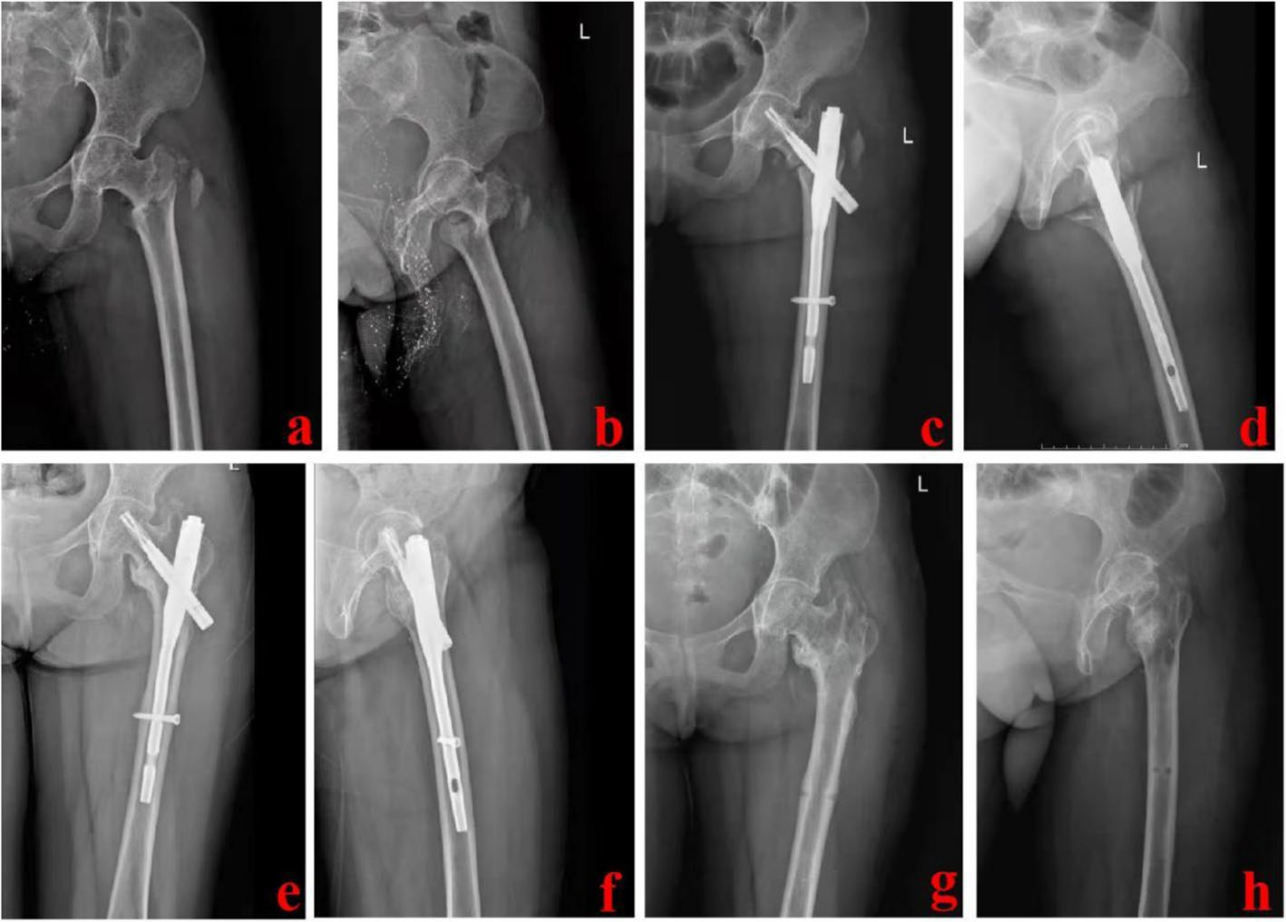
Follow-up image data of the 63-year-old female. a and b AP and lateral images of the hip joint before operation; c and d Postoperative X-ray images showed good reduction and fixation; e and f One year after the operation, the fracture healed well; g and h One and a half years after the operation, the internal fixation device was taken out. AP stands for anteroposterior
